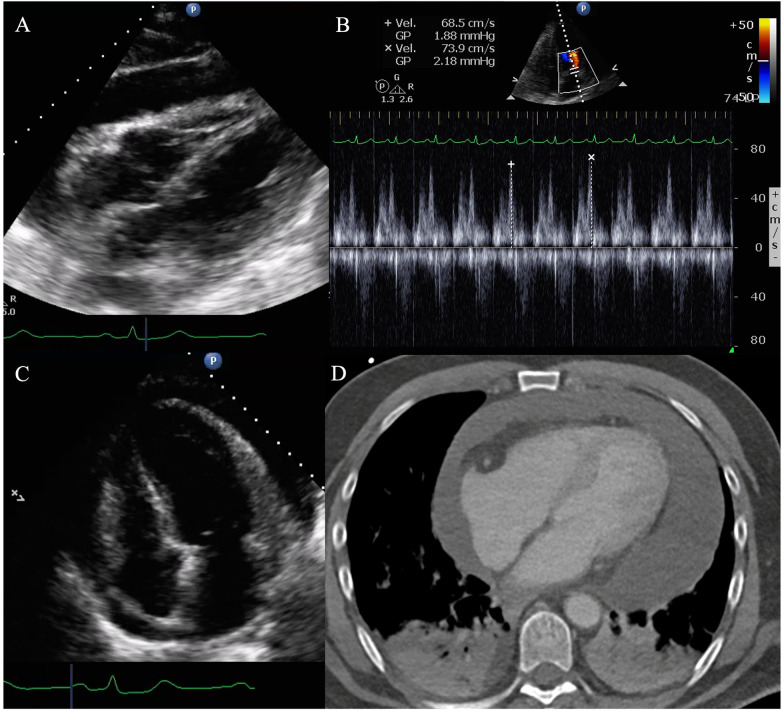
Pericardial effusion on multimodality imaging. (A) Subxiphoid transthoracic echocardiography shows a large circumferential pericardial effusion with reduced left ventricular systolic function. (B) Pulsed-wave Doppler of transmitral inflow demonstrates no significant respiratory variation. (C) Apical four-chamber view confirms a large pericardial effusion. (D) Contrast-enhanced chest computed tomography reveals a large pericardial effusion and concentric thickening of the ascending aortic wall, initially interpreted as an acute aortic syndrome.
